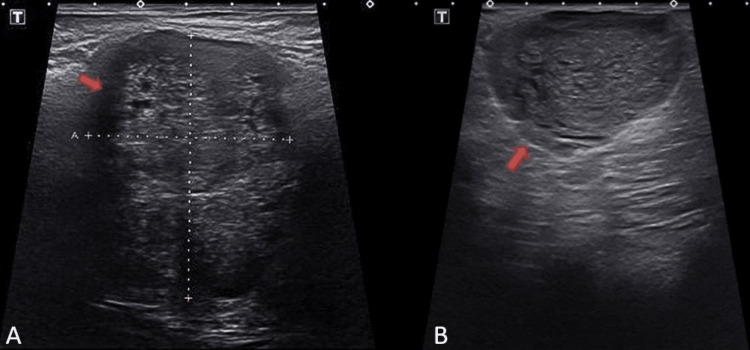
Ultrasound of the left breast indicative of two large masses (red arrows) at the A) 12:00 position and B) 3:00 position, 8 cm from the nipple
